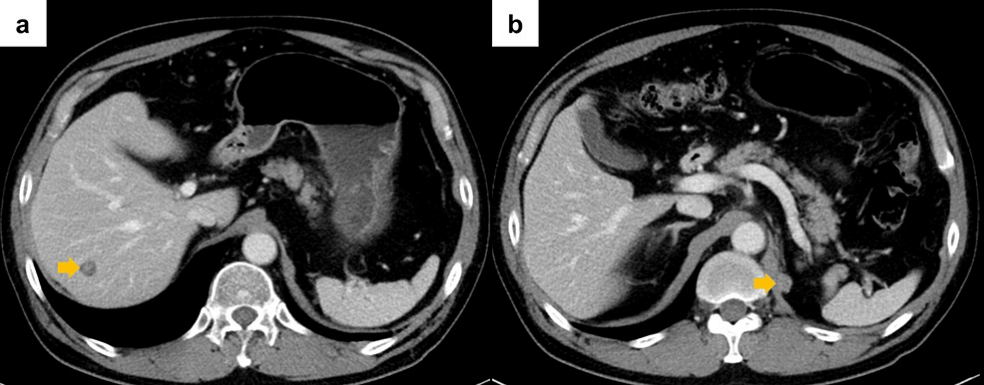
Abdominal computed tomography a) Liver metastasis and b) metastasis to the crus of the diaphragm
